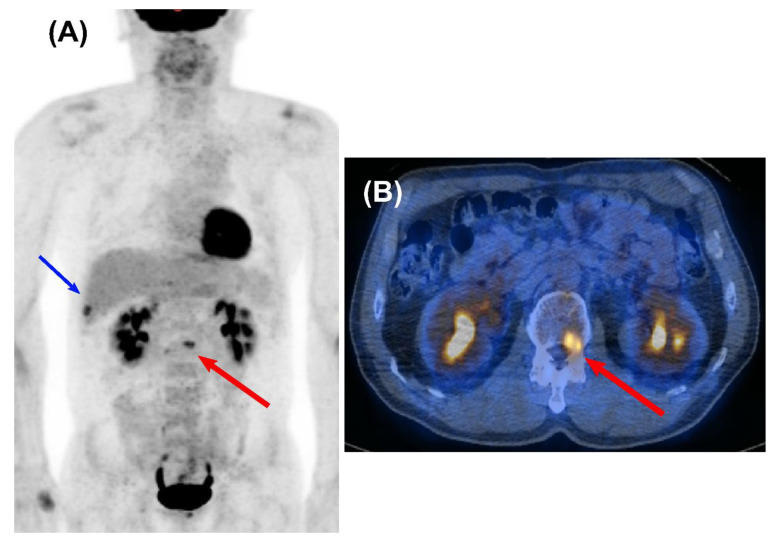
Oligometastasis detected by surveillance FDG PET/CT in a 58-year-old male who underwent curative surgery for NSCLC. A maximum intensity projection (MIP) (A) and a transaxial fused PET/CT image (B) obtained at 16 months after curative resection showed focal FDG uptake on the L2 spine, indicating metastasis to the bone (red arrow). He survived for 70 months after localized radiotherapy with palliative chemotherapy. Another focal FDG uptake on the right lower thorax indicates recent post-traumatic change (blue arrow).
